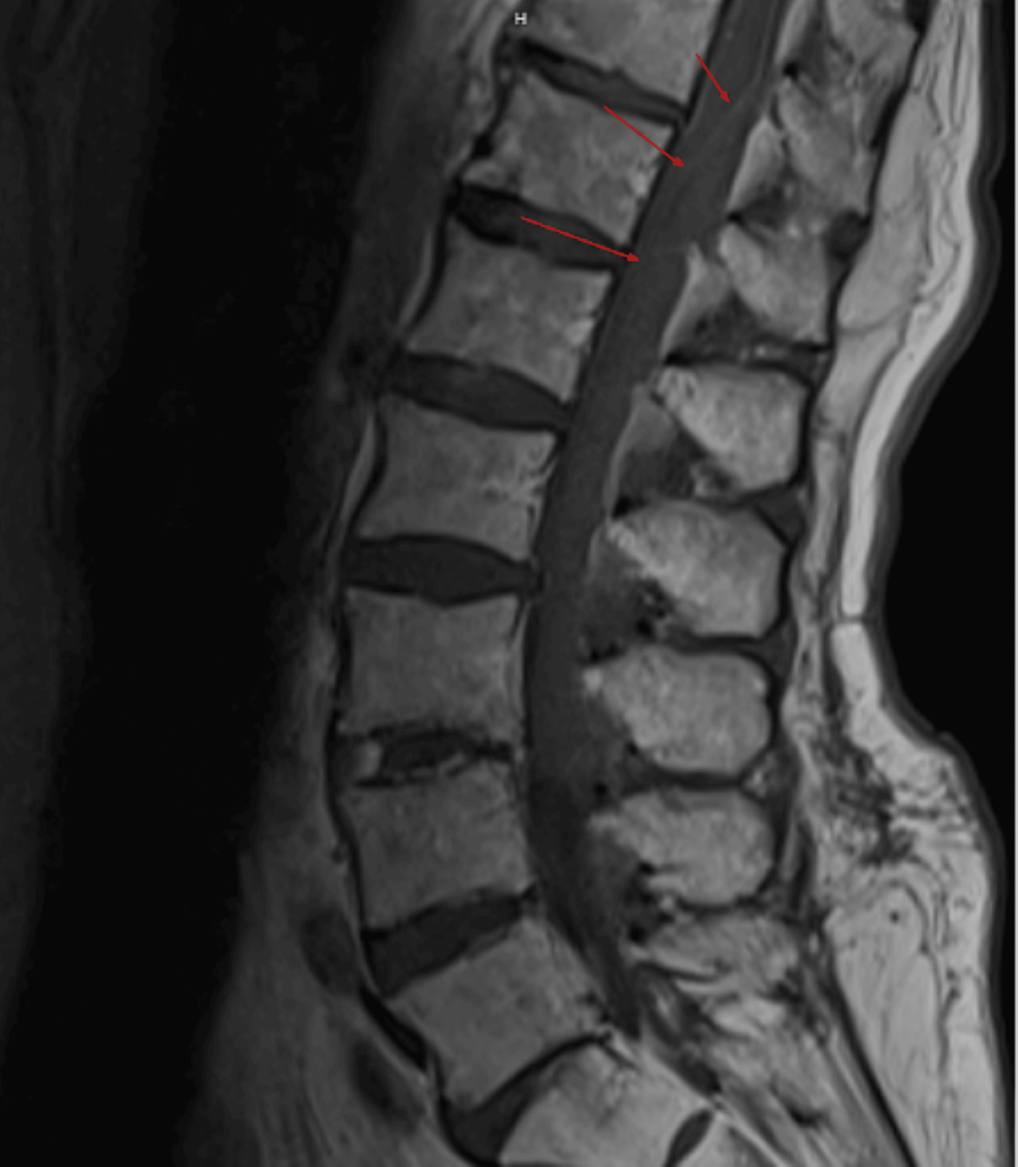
T1 post-contrast sagittal MRI. There is no enhancement of the delineated hyperintense mass within the spinal canal. MRI: magnetic resonance imaging
